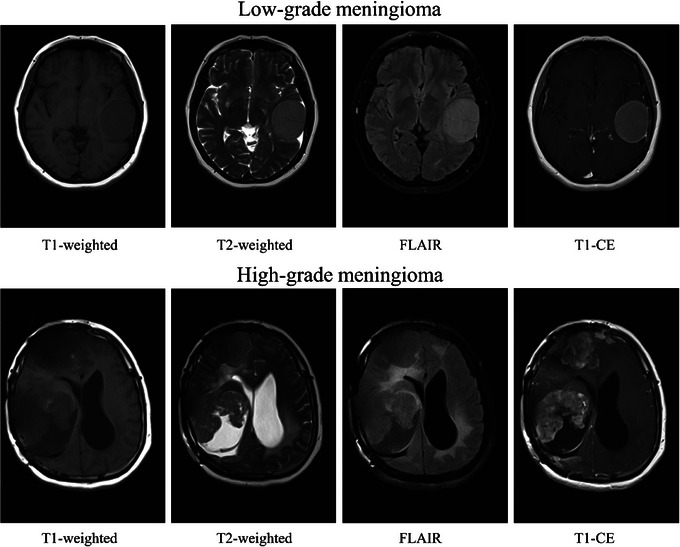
Samples of MR images with low and high‐grade meningiomas.
